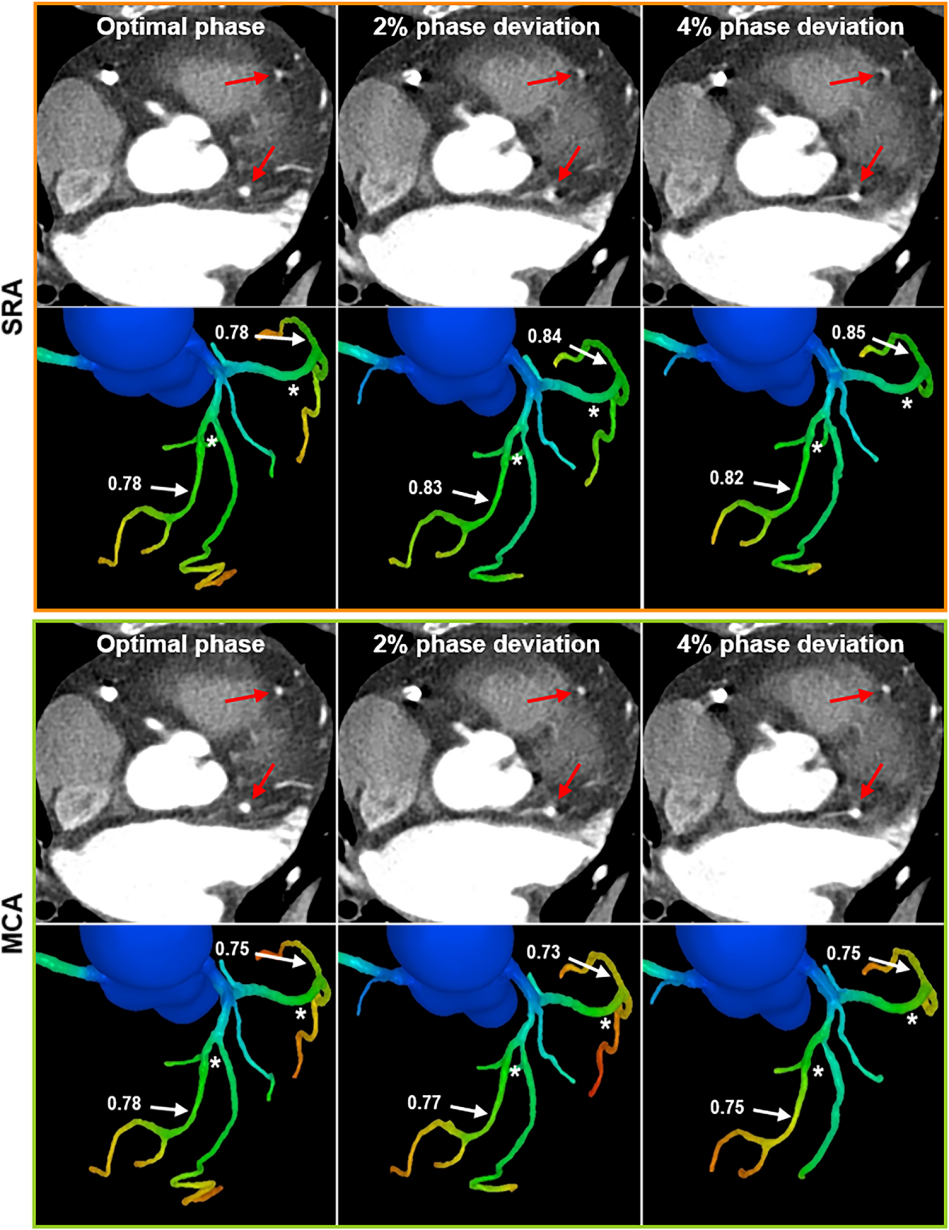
A 75‐year‐old female patient with typical chest pain referred to ICA and invasive FFR measurement following CCTA. ICA confirmed moderate stenosis in the proximal LAD and LCX (white asterisk) with a FFR value of 0.78 and 0.77, respectively. CT‐FFR analysis at the optimal phase (white arrowhead) also revealed that the lesions were functionally significant stenosis. Without MCA, CT‐FFR changed from being significant to nonsignificant (>0.8) at 2 and 4% phase deviation. By applying the MCA, motion artifacts were substantially suppressed (red arrowhead) in the CCTA images and the CT‐FFR at up to 4% phase deviation were kept well consistent with invasive FFR. CCTA, coronary computed tomography angiography; CT‐FFR, CCTA‐derived fractional flow reserve; FFR, fractional flow reserve; ICA, invasive coronary angiography; LAD, left anterior descending artery; LCX, left circumflex artery; MCA, motion correction algorithm.
